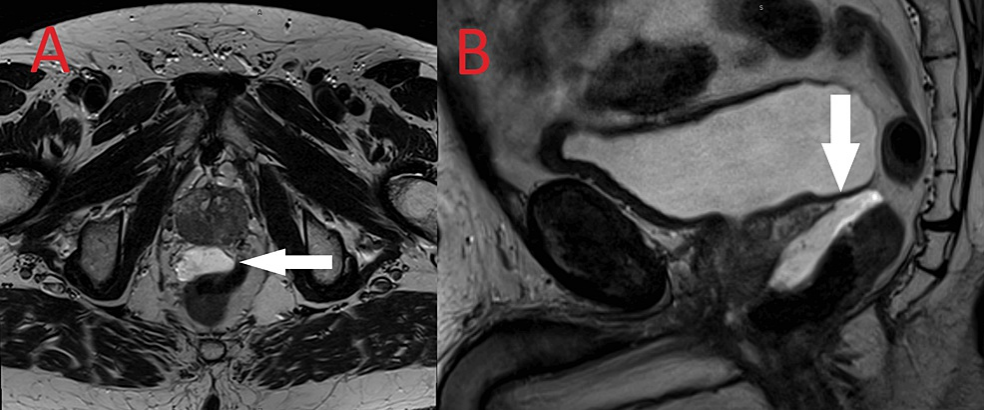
Prostate magnetic resonance imaging is used for radiotherapy planning. A hyperintense T2 signal is seen in the spaceOAR barrier gel inserted between the rectum and prostate. (A) Axial view. (B) Sagittal view.
